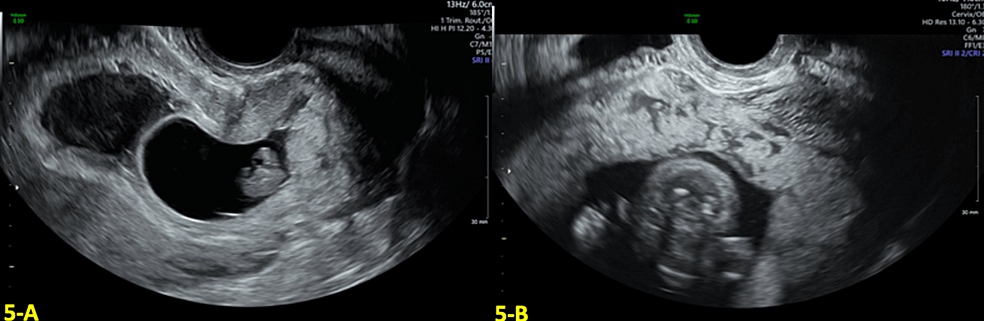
Transvaginal ultrasound (TVUS) findings in the first and second trimesters A: Transvaginal ultrasound (TVUS) at eight weeks and five days gestational age (GA) showed a low-lying gestational sac at the location of the cesarean section (CS) scar. B: Anterior placenta previa with multiple placental lakes at 16 weeks and four days GA.
